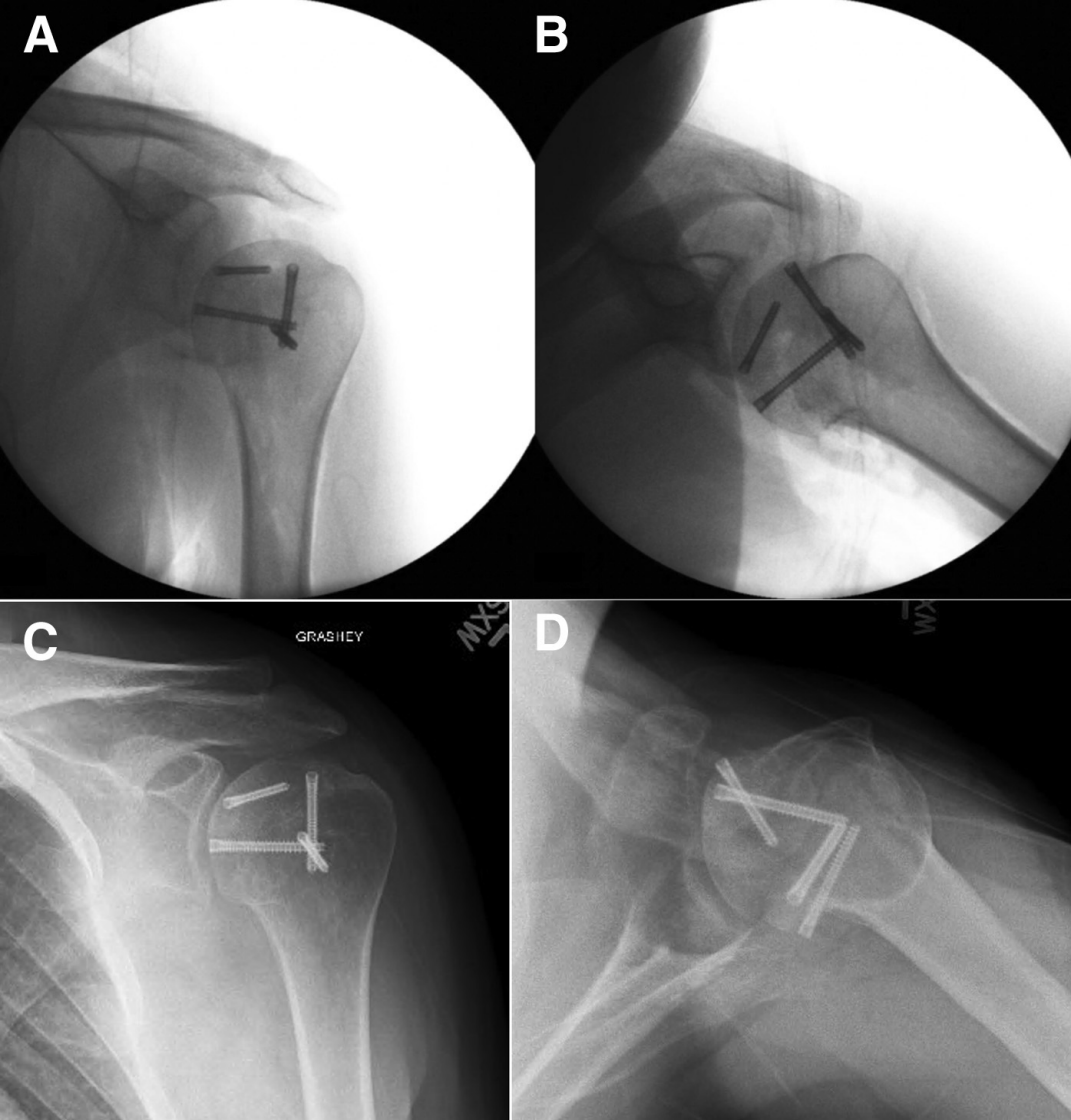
Intraoperative fluoroscopic images and 6-month follow-up radiographs of the left shoulder after completed fixation of osteochondral allograft wedges for reverse Hill–Sachs and Hill–Sachs lesions. (A) Intraoperative anteroposterior image shows the screws recessed below the articular cartilage. (B) Intraoperative axillary lateral view shows restoration of the native humeral head contour and screws recessed below the articular cartilage. (C and D) Six-month postoperative Grashey and axillary lateral radiographs of the left shoulder demonstrating interval healing of the graft without evidence of resorption.
